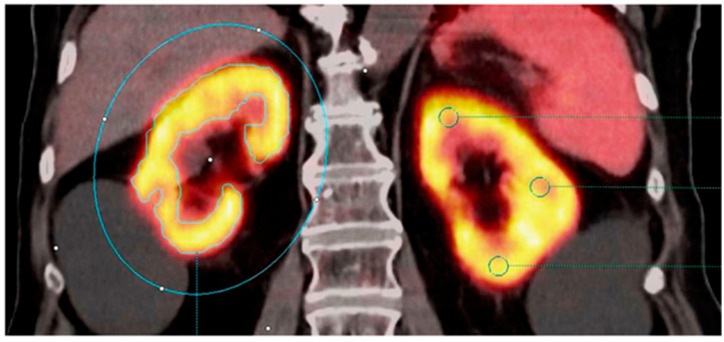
Quantification of renal ^68^Ga-PSMA-11 uptake on frontal PET/CT. Right kidney (left side of image): Whole-kidney VOI with 30% isocontour (blue) for SUV and volume measurements. Left kidney: Three 0.5 cm VOIs (green) for manual SUVmean assessment. Renal cysts without tracer uptake were excluded. This figure is adapted from reference [25], available under a Creative Commons Attribution (CC BY 4.0) license.
